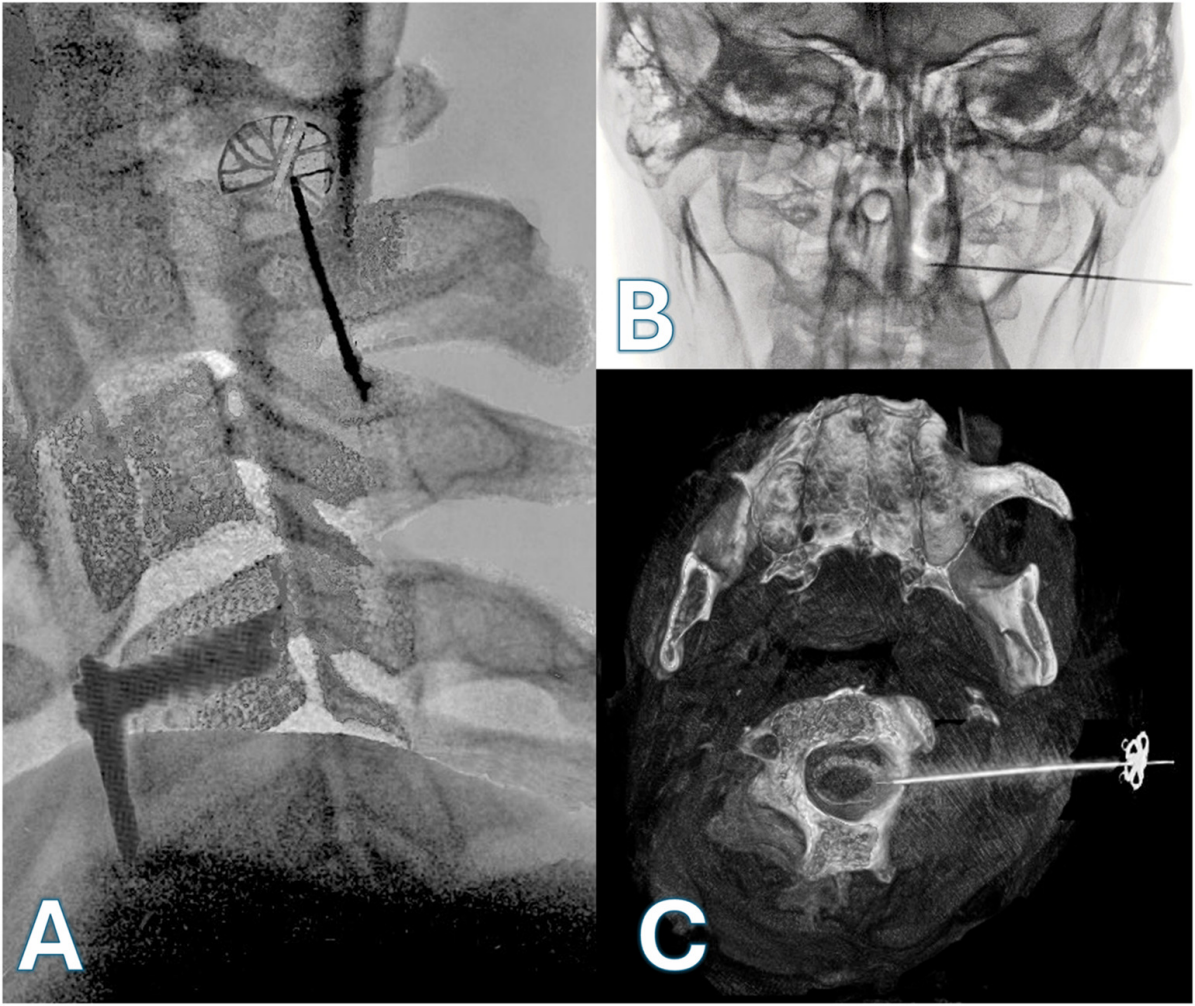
Fluoroscopic and CT-guided imaging of C1–C2 intrathecal access. (A): Lateral fluoroscopic view showing the trajectory of the Tuohy needle through the C1–C2 interlaminar space toward the intrathecal compartment. (B): Anteroposterior fluoroscopic view confirming midline alignment of the needle. (C): Axial CT image following contrast injection, clearly outlining the spinal cord and confirming intrathecal placement.
